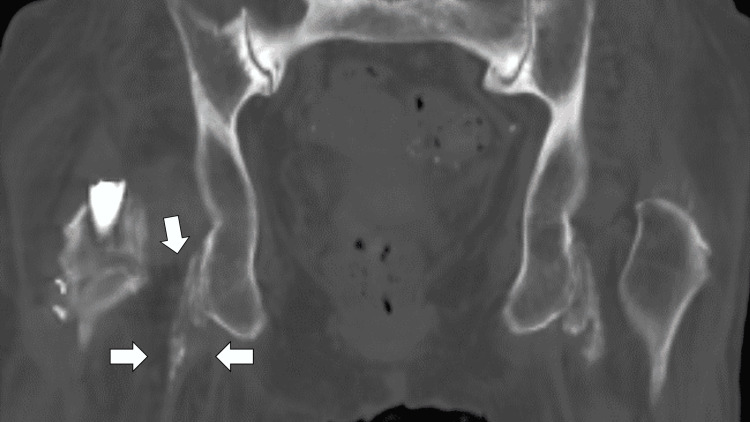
Femoral CT showing multiple migratory calcifications around the right ischial tuberosity surrounding the edematous change of the soft tissues (white arrows) CT: computed tomography
